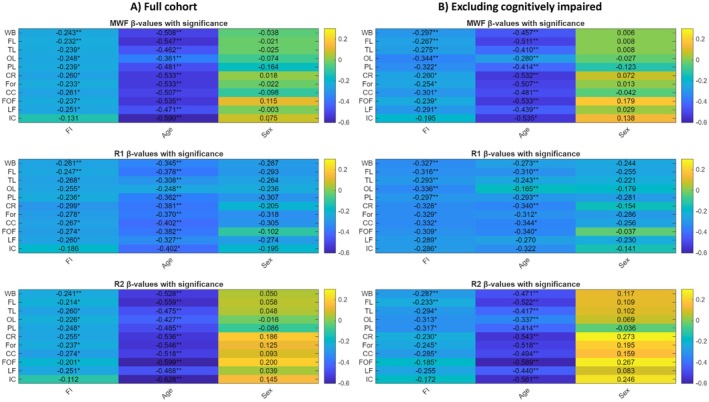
ROI‐wise standardized regression coefficients (β) and *p* values, after FDR correction, for associations between frailty index (FI), age, and sex with regional MRI biomarkers (MWF, R1, and R2) are shown for (A) the full cohort and (B) excluding cognitively impaired participants. Negative β_FI_ values indicate lower myelin content or reduced white matter (WM) integrity with higher frailty. CC, corpus callosum; CR, corona radiata; FL, frontal lobes; FOF, fronto‐occipital fasciculus; For, forceps; IC, internal capsule; LF, longitudinal fasciculus; OL, occipital lobes; PL, parietal lobes; TL, temporal lobes; WB, whole brain; *Indicates *p* < 0.05; indicates *p* < 0.01.
